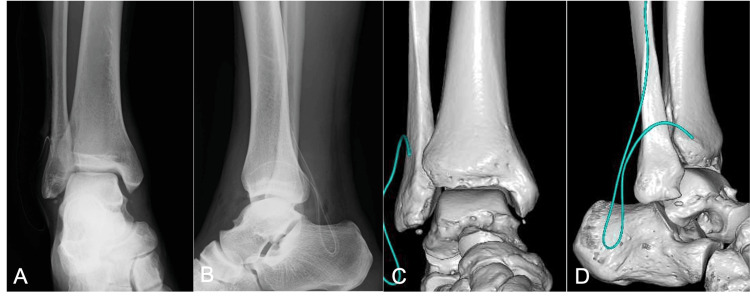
Postoperative plain radiographs and CT scans of the patient's right ankle. Plain anteroposterior (A) and lateral (B) radiographs revealed reduction. The 3D-CT scans (C, D) showed a small bone fragment at the tip of the fibula, which was thought to be an old avulsion fracture.
